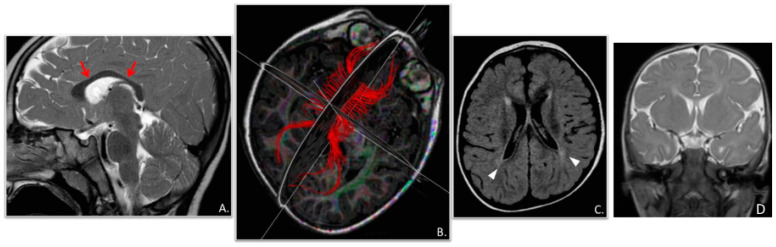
Brain magnetic resonance imaging (MRI) performed at 2.5 years old. (A) A midline sagittal T2-weighted Fast Spin-Echo (FSE) image shows mild corpus callosum (CC) hypoplasia with prevalent involvement of the CC trunk (arrows). (B) Diffusion Tensor Imaging (DTI) Tractography with CC (red color) fiber tracking. (C) An Axial Fluid-Attenuated Inversion Recovery (FLAIR) image displays bilateral paratrigonal white matter hyperintensity (arrowheads). (D) Simplified gyration of the cerebral cortex.
